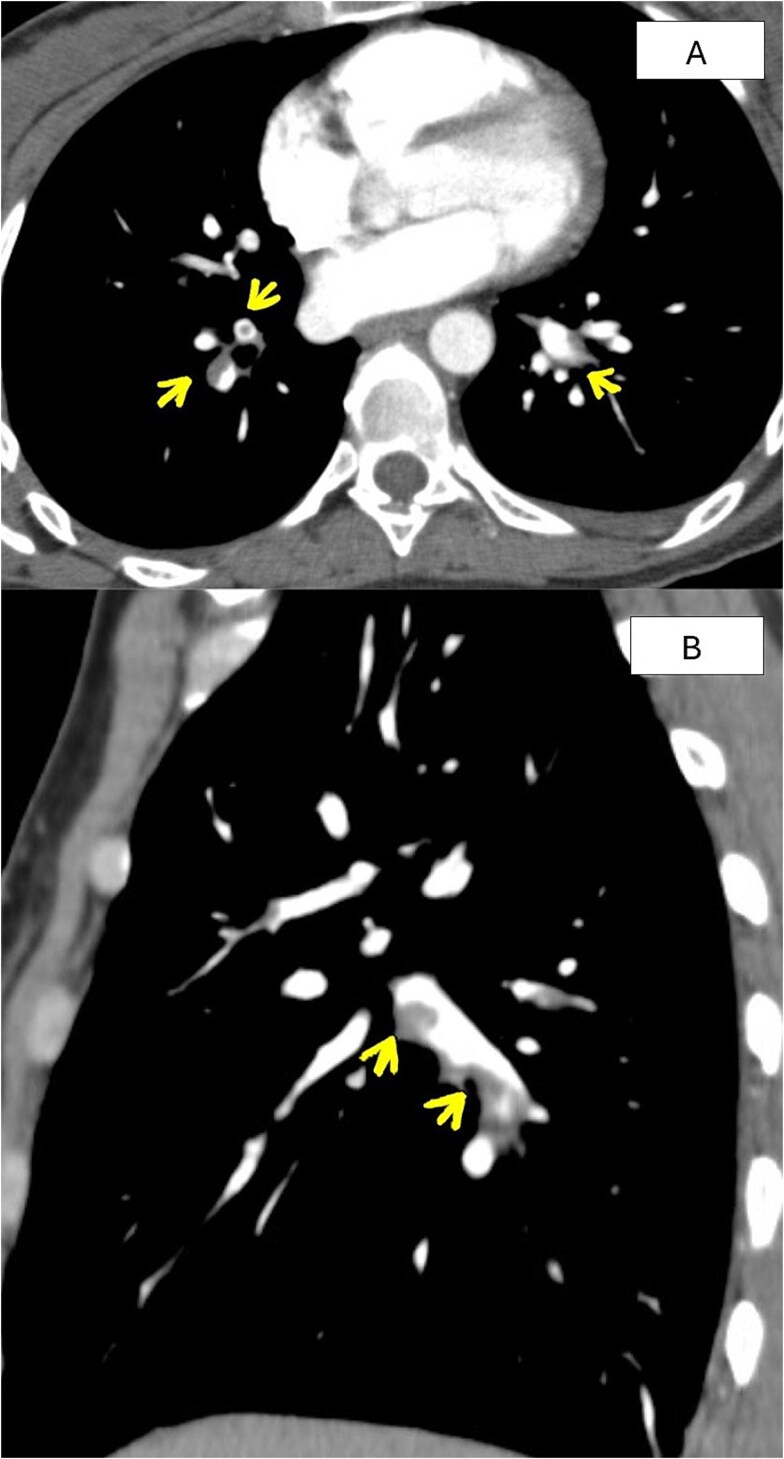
(A) CT angiogram (CTA) chest (august 2020) showing multiple partial thrombi in the right subsegmental left segmental branches (arrowheads). (B) Sagittal 2-dimensional reconstruction CTA chest (august 2020) reveals mural partial thrombosis. Filling defects in the segmental and subsegmental right pulmonary artery branches (arrowheads).
